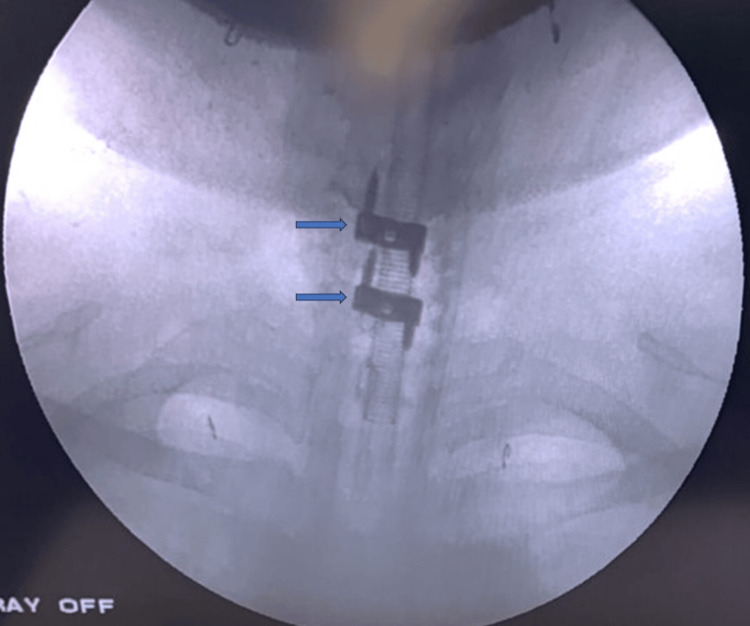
Postoperative X-ray of the cervical spine showing implants at C4/5 and C5/6 (blue arrows)
